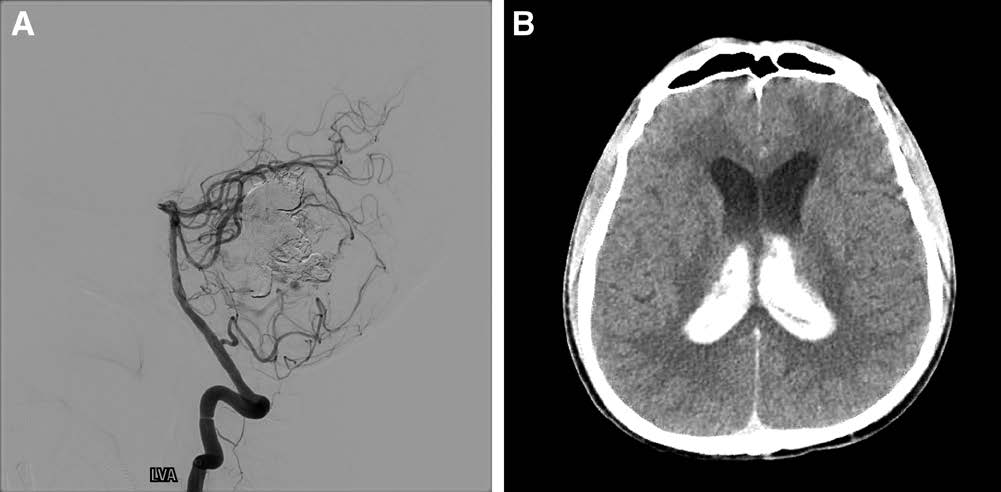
(A) Postprocedural angiogram of the left vertebral artery showed near total obliteration of the arteriovenous malformation (AVM) nidus. (B) Postprocedural head computed tomography (CT) revealed an interventricular hemorrhage in all ventricles with obstructive hydrocephalus.
